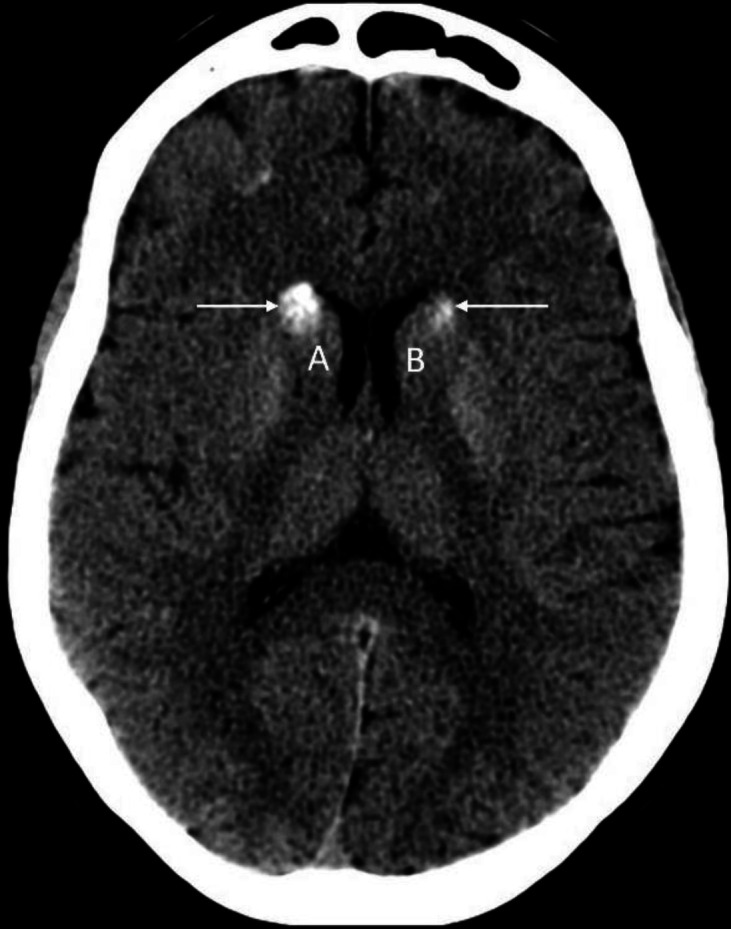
Non-contrast axial CT brain of a 56 years-old male shows calcification (arrows) of the head of caudate nucleus bilaterally (A-B). Calcification in this location and other part of basal ganglia can be seen incidentally in older individuals and generally considered to be of no clinical significance. However, entities like Fahr disease, lead and carbon monoxide poisoning, tuberculosis, neuro-cysticercosis, toxoplasmosis and some metabolic disorders (e.g. hypo and hyperparathyroidism etc.) can also result in calcification
